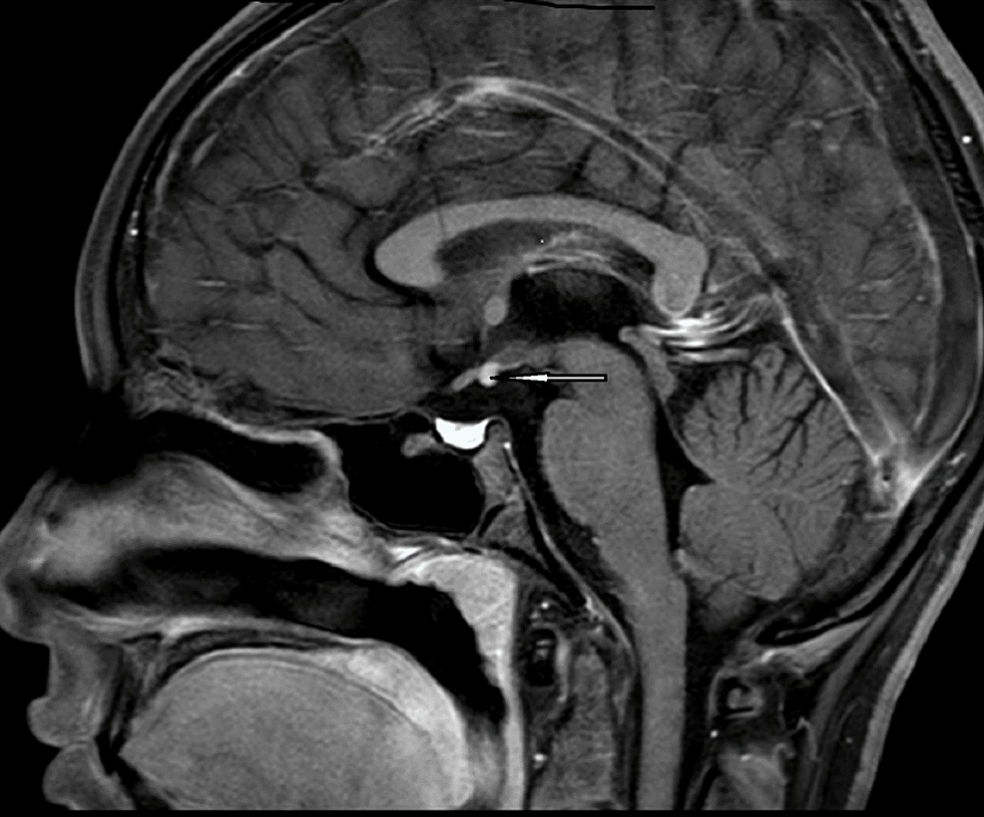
Sagittal post-contrast magnetic resonance imaging of the brain shows an absence of the posterior pituitary in the pituitary fossa The enhancing nodule, visible along the inferior aspect of the optic chiasma, is suggestive of an ectopic pituitary (depicted by a white arrow).
